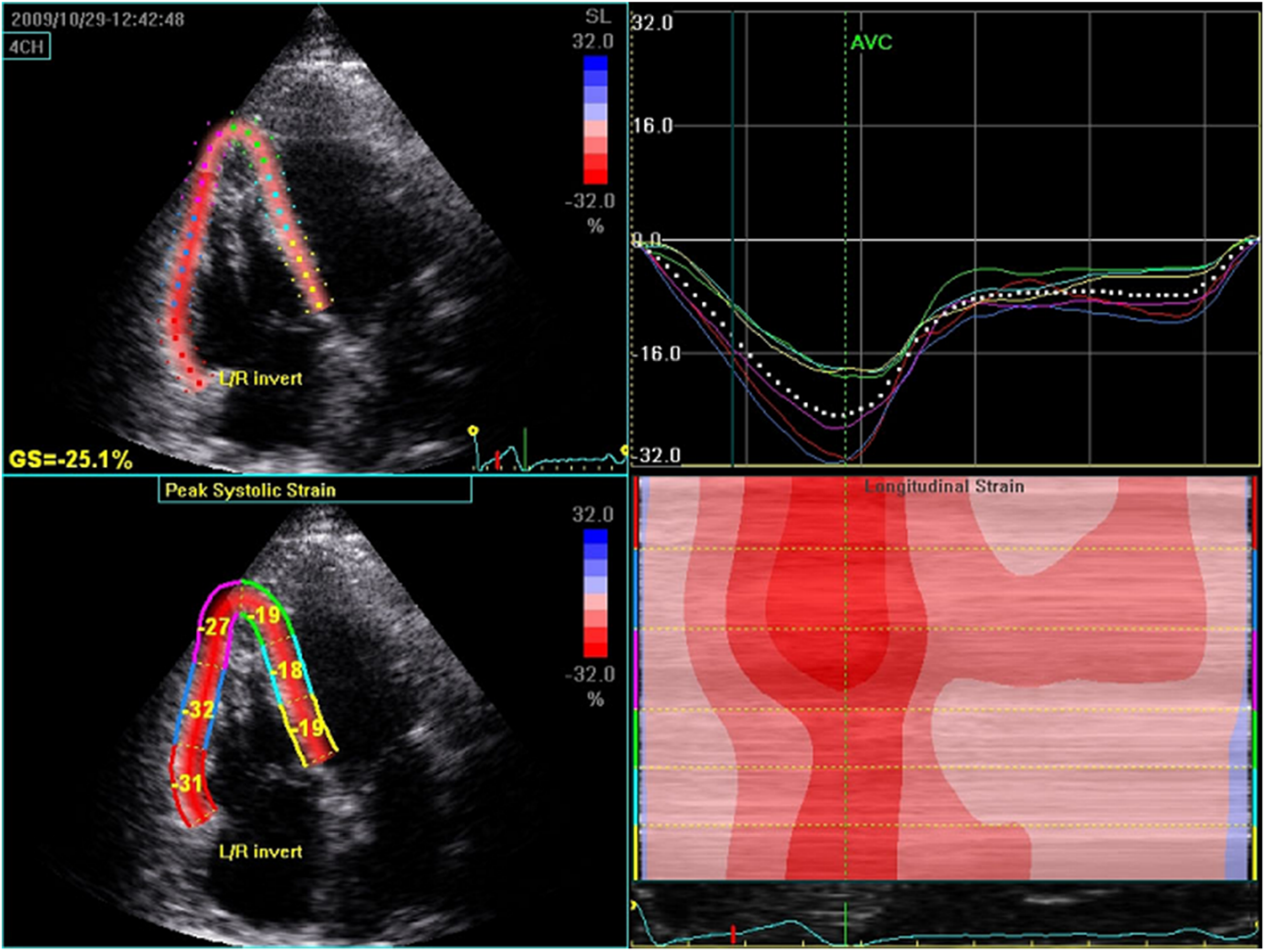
Determination of longitudinal strain in an apical four chamber view. Left side: Colour display of peak systolic strain. The right side shows average segmental strain graphically displayed (upper) and an M-mode representation of peak systolic strain (lower).
